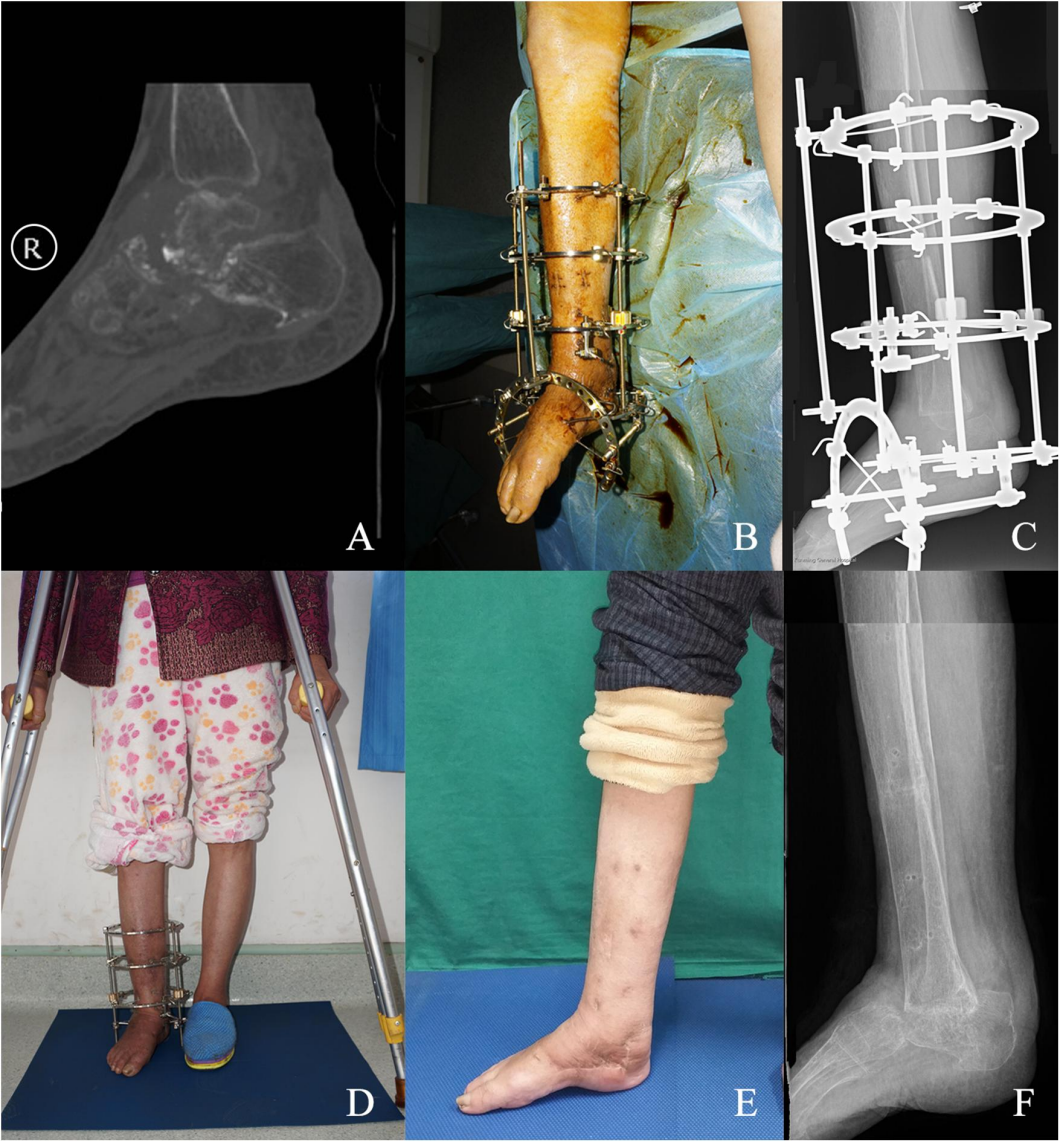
Female patient, aged 58 years: (A) preoperative ankle joint plain CT imaging revealed manifestations of ankle joint tuberculosis and talus necrosis, with the absence of active pulmonary tuberculosis. The preoperative AOFAS score stood at 35 points. (B) Subsequent to a 3-week course of anti-tuberculosis therapy, the patient underwent talus excision surgery, coupled with partial resection of the distal tibia until achieving normal, uninfected bone integrity. Following meticulous wound debridement, a minimally invasive midshaft tibial osteotomy and tibiocalcaneal fusion procedure were performed. (C) Postoperatively, radiographic assessment of the lower limb unveiled a mid-lower segment tibial transection, with the application of an Ilizarov external fixator in a state of bone traction. (D) Postoperative ambulation was initiated with the aid of crutches, alongside participation in structured rehabilitation programs, and a continuation of anti-tuberculosis treatment for a duration of six months. A subsequent three-month follow-up x-ray evaluation revealed favorable tibio-calcaneal fusion and commendable bone mineralization in the midshaft of the tibia, prompting the removal of the external fixator. (E) A comprehensive two-year follow-up examination demonstrated the patient's report of enhanced lower limb mobility and sensory perception, accompanied by an elevated AOFAS score of 84 points.
